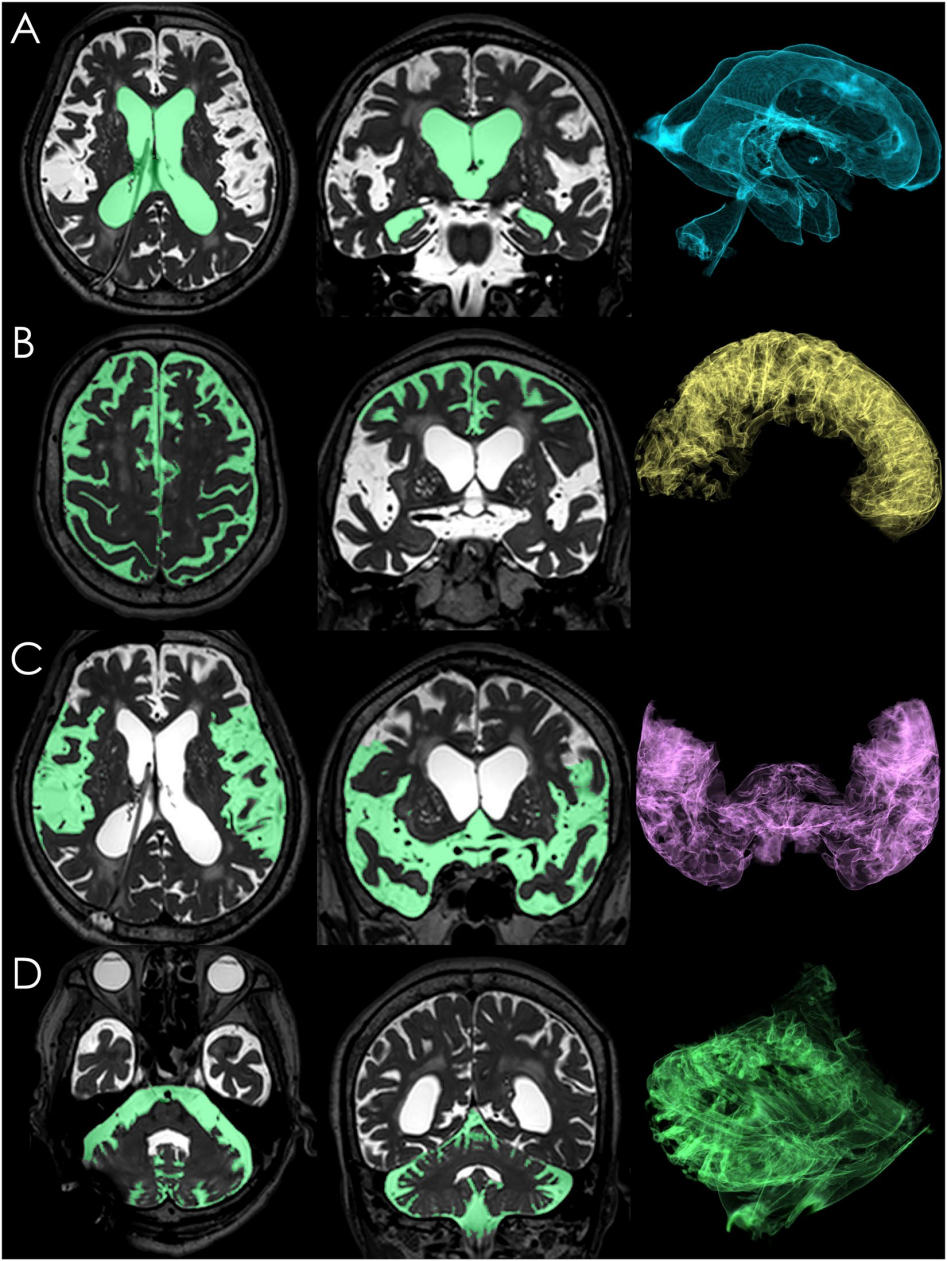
Segmentation of the total ventricles and three parts of the subarachnoid spaces. These images were created on the SYNAPSE 3D workstation (Fujifilm Medical Systems, Tokyo, Japan) from a three-dimensional (3D) T2-weighted 3-Tesla sequence 10 days after ventriculoperitoneal shunting via parieto-occipital approach in a patient diagnosed with co-occurrence of idiopathic normal pressure hydrocephalus (iNPH) and Alzheimer’s disease (AD). The ventricles were manually extracted from intracranial cerebrospinal fluid (CSF) space by enclosing in a free shape. (A) The total subarachnoid spaces were segmented by subtraction of the total ventricles from total intracranial CSF spaces. The convexity subarachnoid space (B) was manually segmented from the total subarachnoid spaces per the anatomical landmarks of the basal interhemispheric cistern, Sylvian fissure, and tentorium cerebelli. From the residual subarachnoid spaces, the subarachnoid space in the posterior fossa (D) was segmented with reference to the anatomical landmarks of the tentorium cerebelli, chiasma and optic tract. There was a small MRI artefact in the right occipital posterior fossa. The Sylvian fissure and basal cistern (C) were segmented by subtraction of the convexity subarachnoid space and subarachnoid space in the posterior fossa from the total subarachnoid spaces. The convexity subarachnoid space per ventricle ratio (CVR), which was defined as the volume of the convexity subarachnoid space (178 mL) divided by the total ventricular volume (118 mL), was 1.51.
